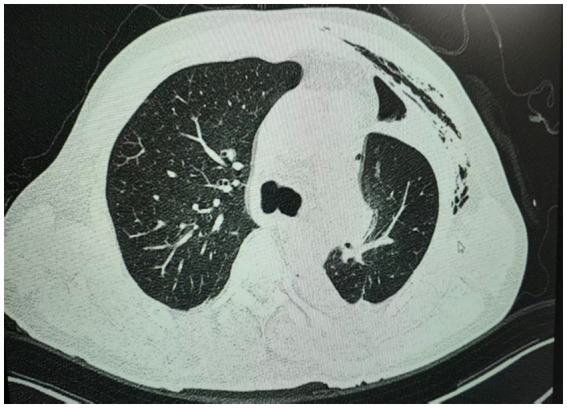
Postoperative computed tomography (CT) scan on day 2 after the surgery during the first hospitalization showed full expansion of the residual lung without evidence of pneumothorax or parenchymal compression.
